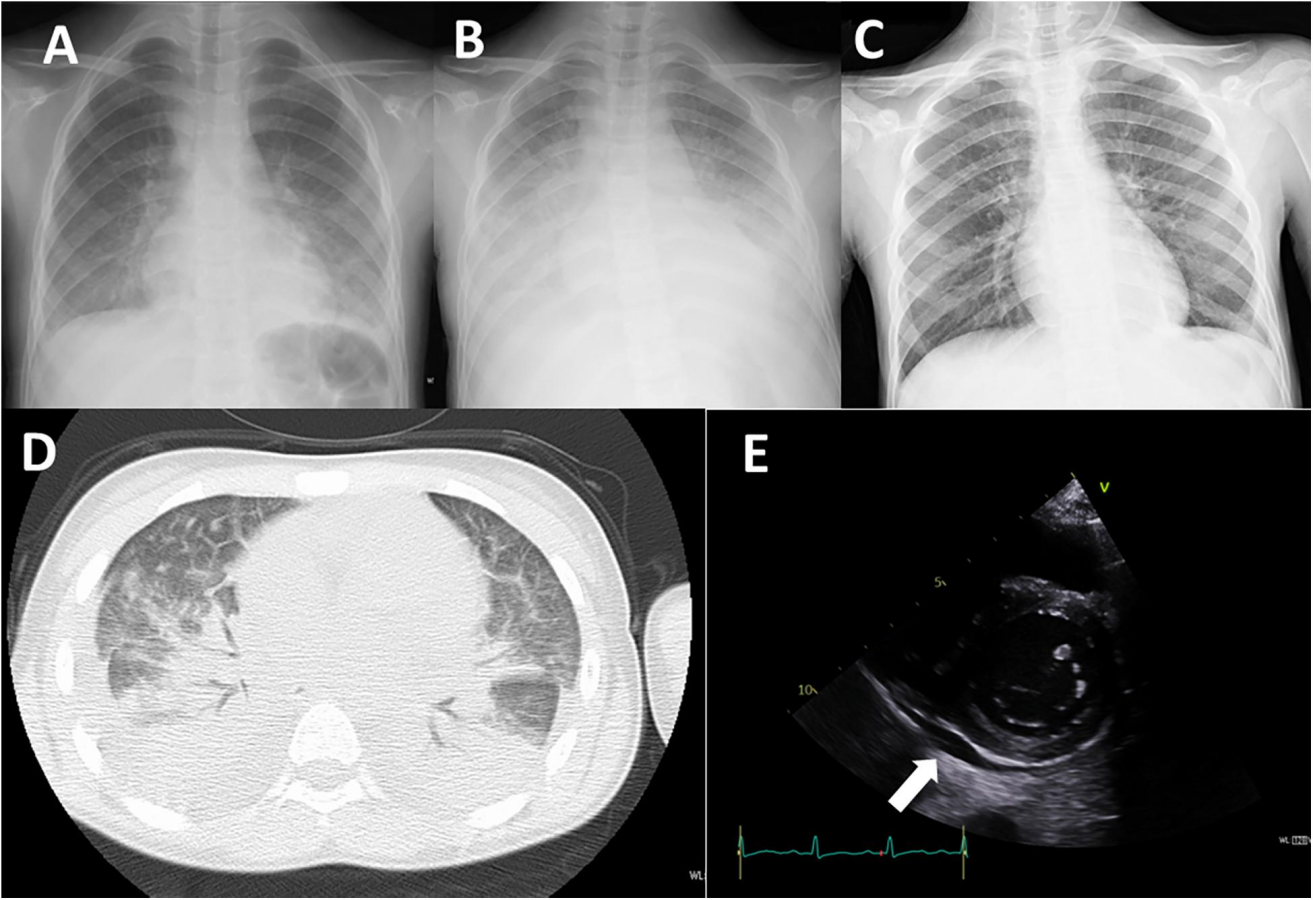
Imaging studies. (A) Chest x-ray on admission to the previous hospital. (B) Chest x-ray on admission to our hospital (day 7 of illness). (C) Chest x-ray on day 12 of treatment (day 19 of illness). (D) Chest CT image on admission to our hospital (day 7 of illness). E: Echocardiography on admission to our hospital (day 7 of illness). Arrow indicates pericardial effusion.
